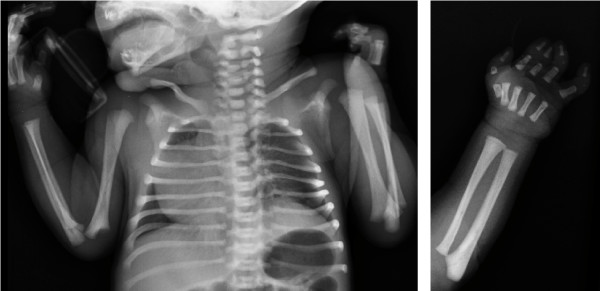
Chest X-ray: asymmetric chest with reduction of the third to fifth left intercostal spaces and dextrocardia (2a). Left hand X-ray: hypoplasia of the main phalanx of the I finger, absence of the intermediate phalanx of the II and III fingers, and substitution of the intermediate phalanx of the IV and V fingers with a small ossification nucleous (2b).
